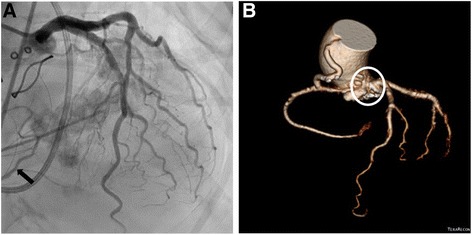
Postoperative imaging. a Coronary angiography images on postoperative day 4. The origin of the right coronary artery was not observed; however, the distal right coronary artery was seen because of backflow (*arrow*). b Computed tomography coronary angiography images. The coronary artery bypass graft was observed from the ascending aorta to the proximal right coronary artery, and the proximal right coronary artery was not visible (*white circle*)
